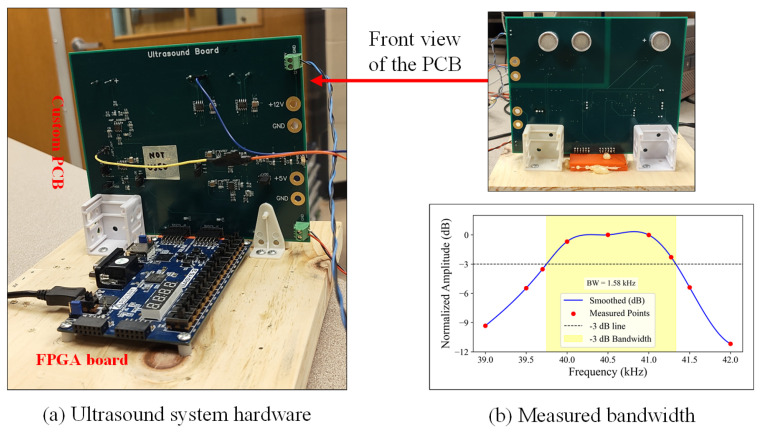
Developed ultrasonic ranging system.
